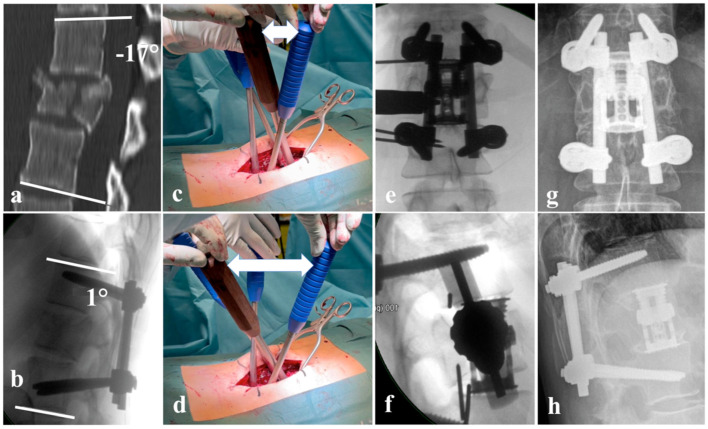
(a) A4, N0 fracture of L1 according to AOSpine classification with relative spinal canal narrowing and 17° fracture-related kyphosis; (b) lateral intraoperative X-ray after fracture reduction to 1° lordosis; (c) position of the reduction levers before lordotic reduction; (d) position of the reduction levers after reduction; the white double arrow marks the increase in distance from (c–f) intraoperative X-ray showing the positioning of the distractible vertebral body replacement cage; (g,h) 15-year follow-up with unchanged position of the fused segments.
